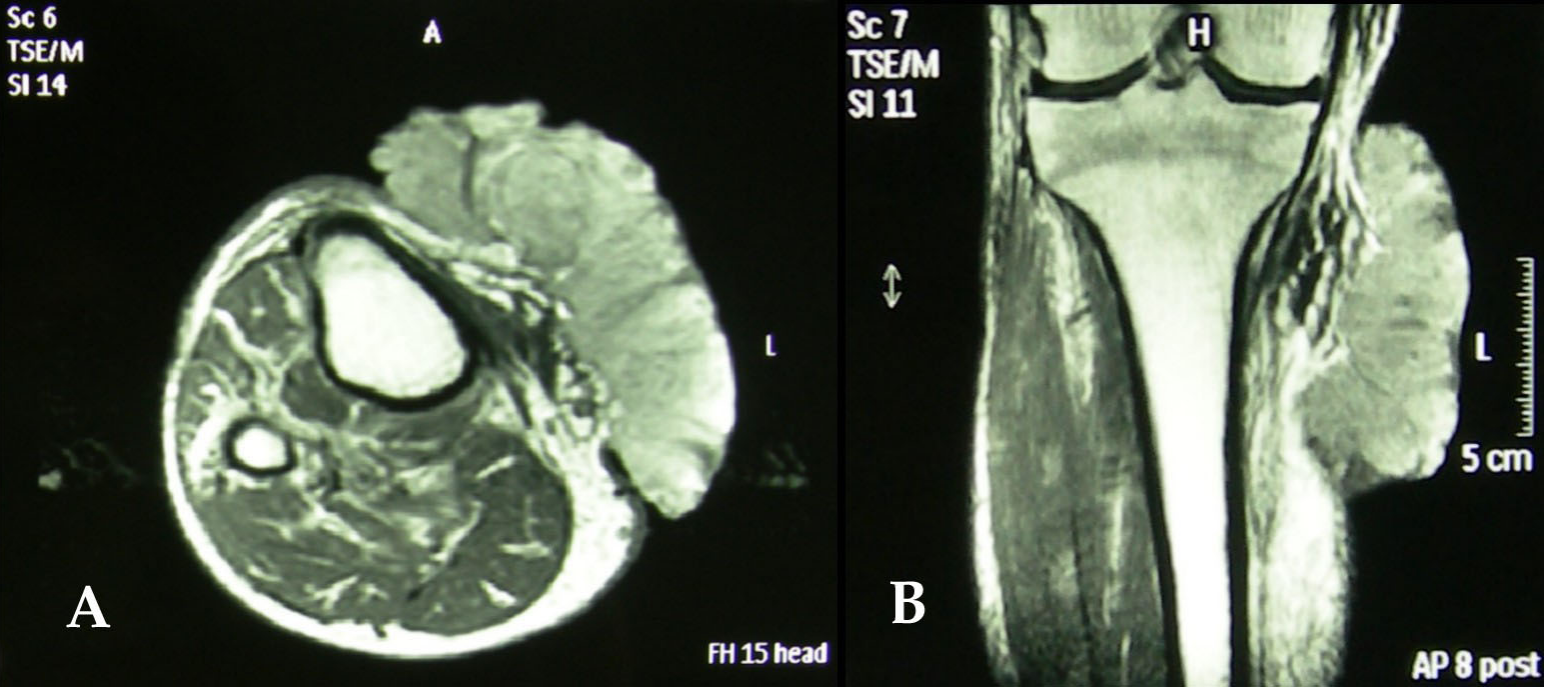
Axial (A) and Coronal (B) MRI T1-weighted image of the right proximal tibia. A large homogeneous mass which was invaded the adjacent soft tissue and extended along the medial surface of cutaneous plane was visible. No signs of bone tissue infiltration, or abnormal intensity in muscle tissues could be seen.
